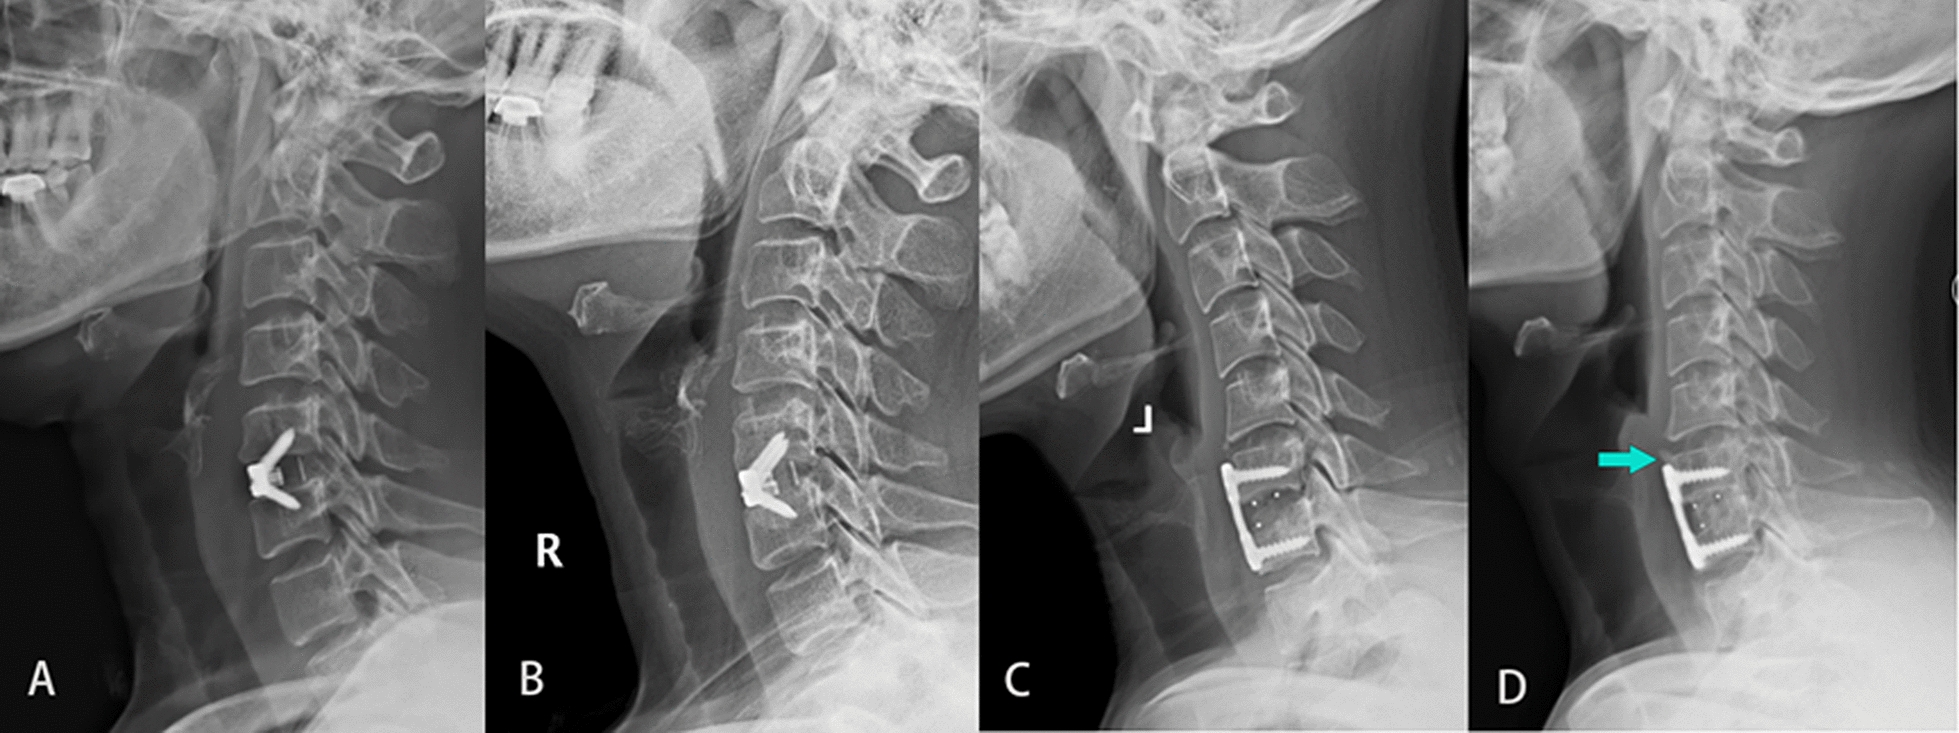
A and C Lateral X-ray image of the cervical spine at 3 months postoperatively. B and D Lateral X-ray images of the cervical spine at 12 months postoperatively. A new anterior bony spur has formed from the tip of the plate to the upper adjacent level, as shown in 2D
